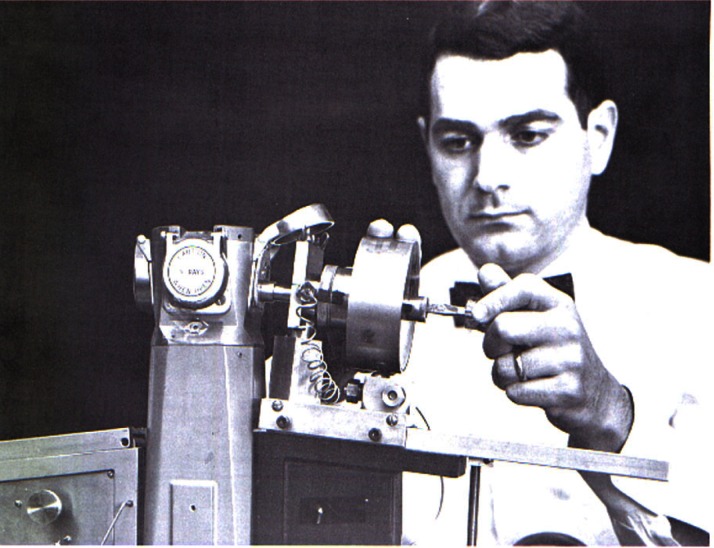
A photograph, taken at NBS in 1964, of the hydraulically-loaded high pressure x-ray powder camera mounted on the table of an x-ray unit. This is the improved version of the design shown in Fig. 3. The film cassette is being adjusted by G. J. Piermarini.
